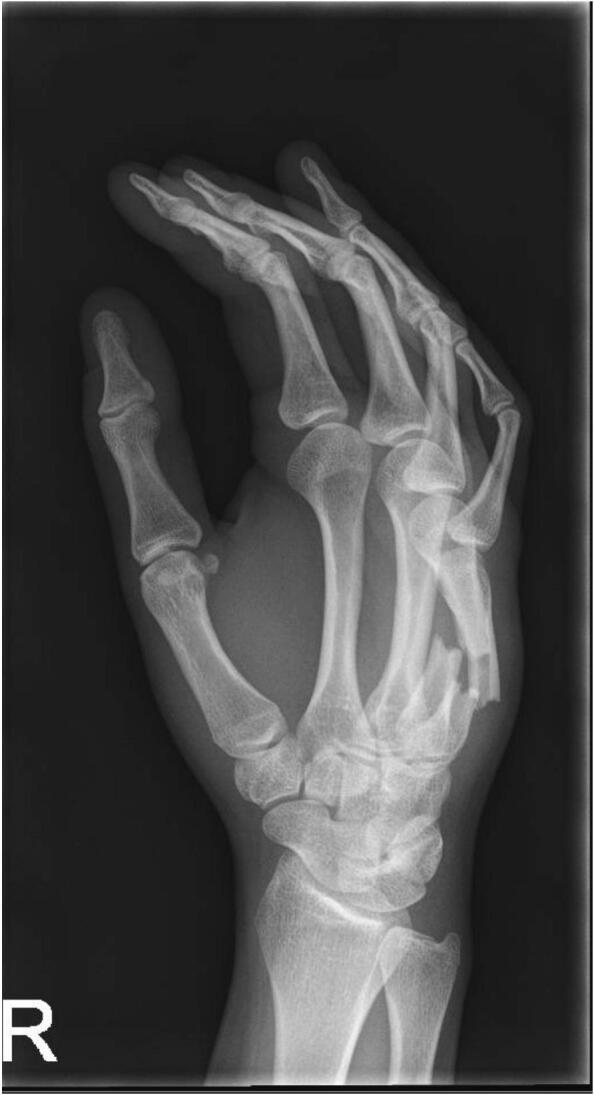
Displaced midshaft fractures of metacarpals IV and V at initial presentation on the Emergency Department, shown on a three-quarter radiograph view.
